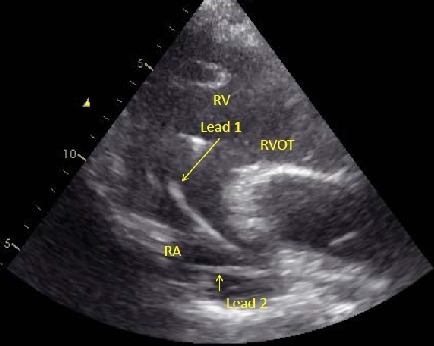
Parasternal short axis view on 2D echocardiogram showing lead 1 across the tricuspid valve into RV outflow while lead 2 is seen in RA.
